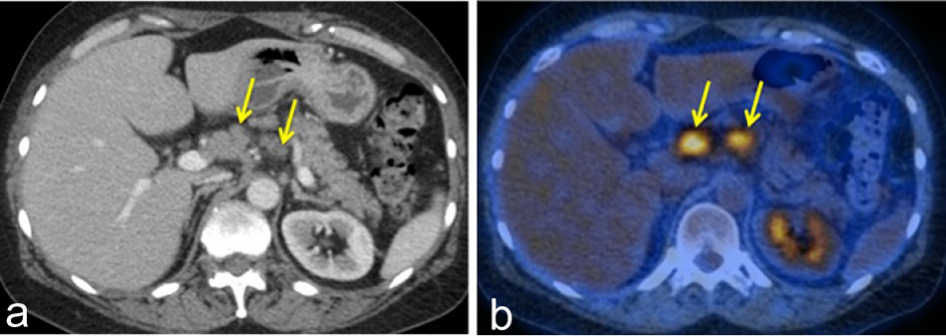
A 35-year-old female with Grade3SCC of the cervix. (a) Demonstration of borderline lymph nodes, suspicious of metastatic disease but at an unusual site in cervical cancer (arrows). The ^18^F-FDG PET CT (b) was performed for further evaluation and demonstrates highly FDG avid lymph nodes, laproscopically confirmed as metastatic SCC lymph nodes (arrows). FDG,fluoro-2-deoxy-D-glucose; ^18^F-FDG PET CT, [fluorine-18]-fluoro-2-deoxy-D-glucose positron emission tomography; SCC, squamous cell carcinoma.
